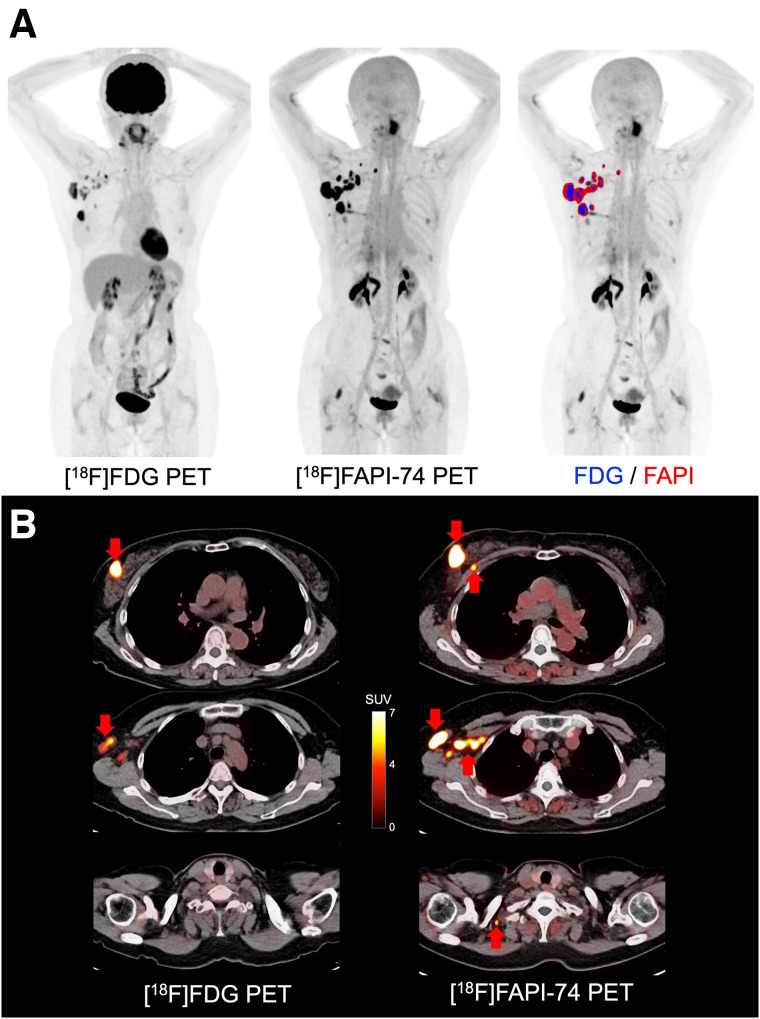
A 59-y-old woman with multiple LN metastases of breast cancer. (A) Comparison of maximum-intensity projection images: image on right shows fusion with positive lesions on [^18^F]FAPI-74 PET (red area) and [^18^F]FDG PET (blue area), (B) PET/CT images on [^18^F]FAPI-74 PET and [^18^F]FDG PET (arrows indicate metastatic lesions). [^18^F]FAPI-74 PET detected more metastatic lesions than [^18^F]FDG PET (SUV_max_ of primary lesion is 19.6 and 13.0, respectively).
